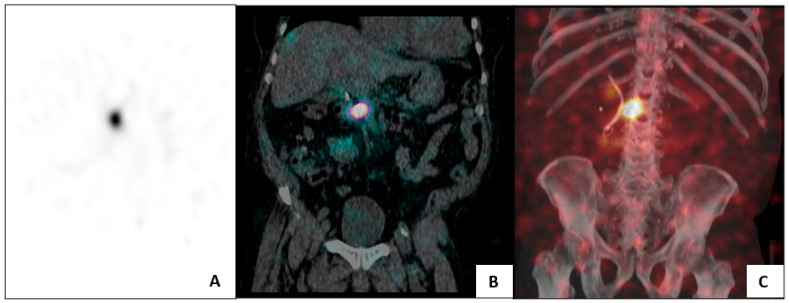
Bremsstrahlung imaging (A) and SPECT-CT scan (B,C) in patient after Oncosil implantation within 4 h to confirm localization and retention of a β-emitting isotope within the pancreas. These images from our cohort demonstrate that the ^32^P isotope has remained in the head of the pancreas with no ectopic distribution.
